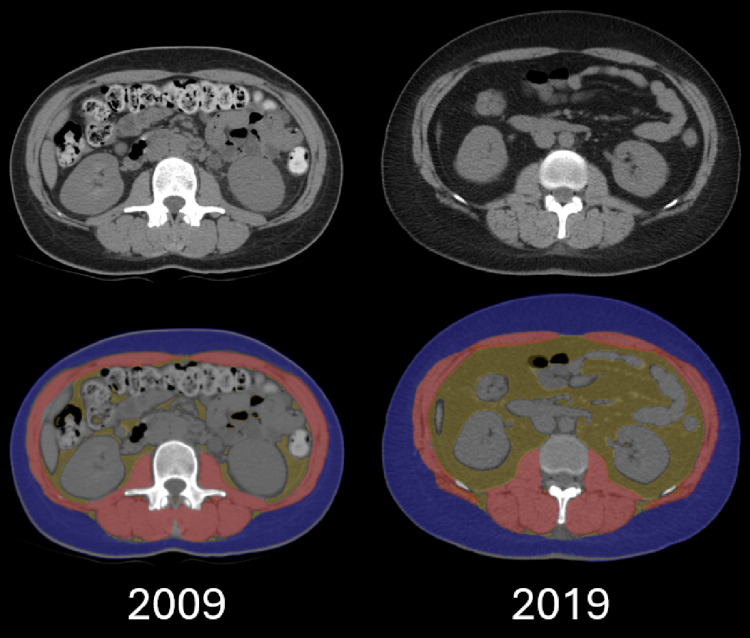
Change in skeletal muscle and abdominal adipose tissue over a 10-year interval. Change in skeletal muscle and abdominal adipose tissue in a 36-year-old female who underwent non-contrast CT of abdomen in 2009 (left column) and again in 2019 (right column) at age 47. Axial slices are shown at the L3 level for both time points, including original CT images (top row) and images showing the fully automated segmentation of skeletal muscle (red), visceral adipose tissue (VAT, yellow), and subcutaneous adipose tissue (SAT, blue). There are visually apparent increases in VAT and SAT area with subtler apparent decreases muscle and VAT density. Change in muscle area is more difficult to assess visually but appears similar. AI-measured skeletal muscle density decreased from 45 Hounsfield units (HU) to 28 HU, for a net decrease of −17 HU over a 10.5-year interval and a rate of −1.7 HU/year. AI-measured skeletal muscle area increased from 110 cm^2^ to 142 cm^2^, for a net increase of 32 cm^2^ and a rate of 3.1 cm^2^/year. AI-measured VAT density decreased from −68 HU to −95 HU, for a net decrease of −27 HU and a rate of −2.7 HU/year. AI-measured VAT area increased from 38 cm^2^ to 211 cm^2^, for a net increase of 173 cm^2^ and a rate of 16.5 cm^2^/year. AI-measured SAT area increased from 113 cm^2^ to 316 cm^2^, for a net increase of 203 cm^2^ and a rate of 19.4 cm^2^/year. Calculated VAT-to-SAT ratio (VSR) increased from 0.34 to 0.67, for a net increase of 0.33 and a rate of 0.03/year. At both time points, the CT indication was to evaluate for kidney stones in the setting of flank pain
